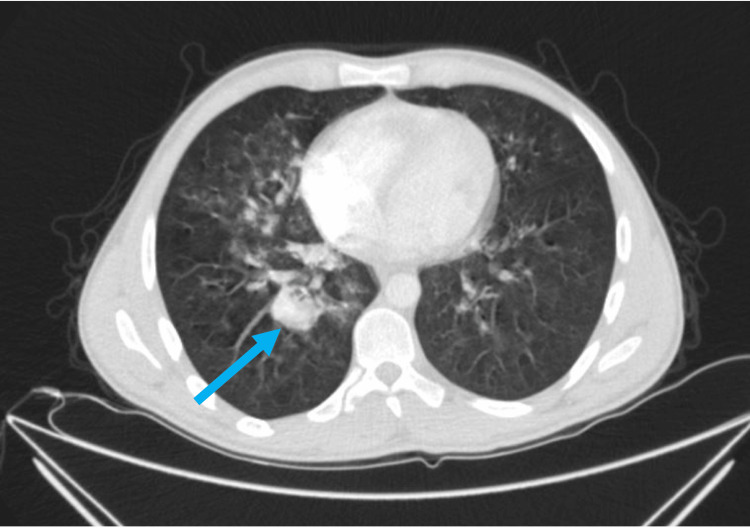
A chest CT scan with the blue arrow showing the pulmonary artery aneurysm (PAA) 2*3 cm
